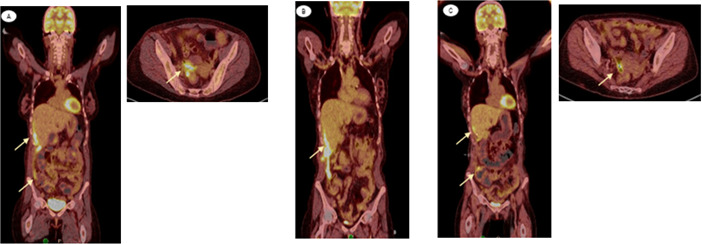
Trend in positron emission tomography/computed tomography (PET/CT) through course, with arrows showing areas of concern (A) Initial PET/CT obtained a month after laparoscopic abscess drainage showing heterogeneous, markedly avid fluorodeoxyglucose (FDG) uptake in the right perihepatic collection extending along the anterior abdominal wall musculature and right peritoneum (left), with additional focal uptake in the midline superior and right pelvis (right). (B) Repeat PET/CT 2 months after laparoscopic drainage, while on cefiderocol and minocycline, showing right liver margin uptake with a new contiguous collection extending into the right lower quadrant and focal thickening/fluid buildup. (C) PET/CT 1 month after right hepatic segment VI resection with cholecystectomy and mobilization of the colon from the abdominal wall showing fluid in the resection cavity measuring approximately 5.8 × 1.3 cm in the axial plane with FDG uptake surrounding the fluid collection and extending superiorly and anteriorly along the right hepatic capsule. Focus of FDG uptake in the right pelvis is decreased compared with prior imaging and was previously considered physiologic bowel activity, though residual inflammation and/or infection related to the prior tubo-ovarian abscess could not be excluded.
